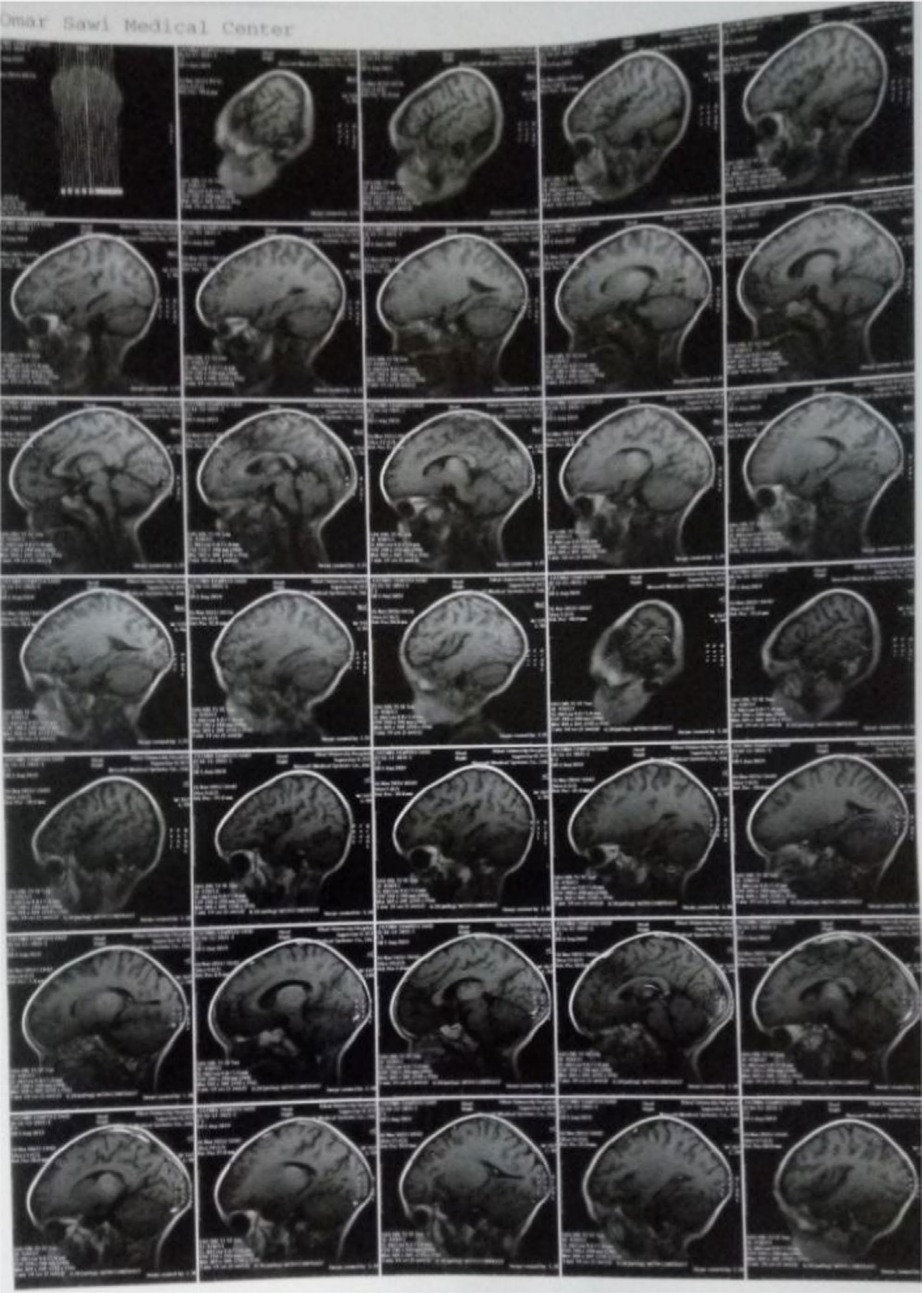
MRI reveals bilateral thalamic lesion due to viral encephalitis or vascular insult
